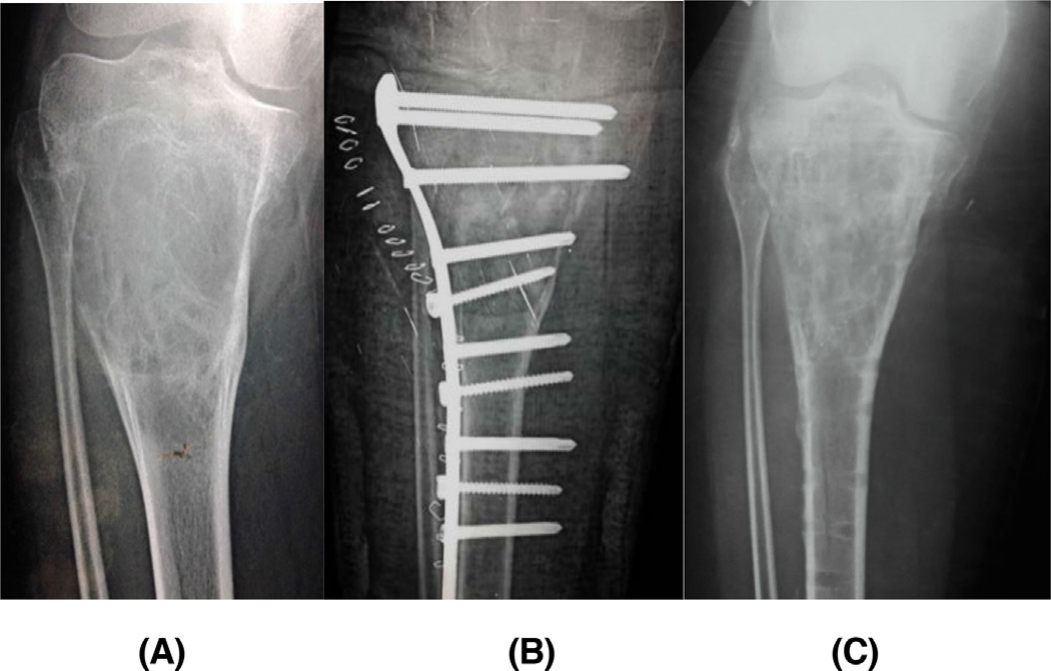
(A) Plain radiograph showed varus mal-alignment of recurrent ABC in the proximal tibia (case 16), (B) post-operative radiograph after revision surgery, and (c) last follow-up radiograph 32 months post-operative showed complete healing.
